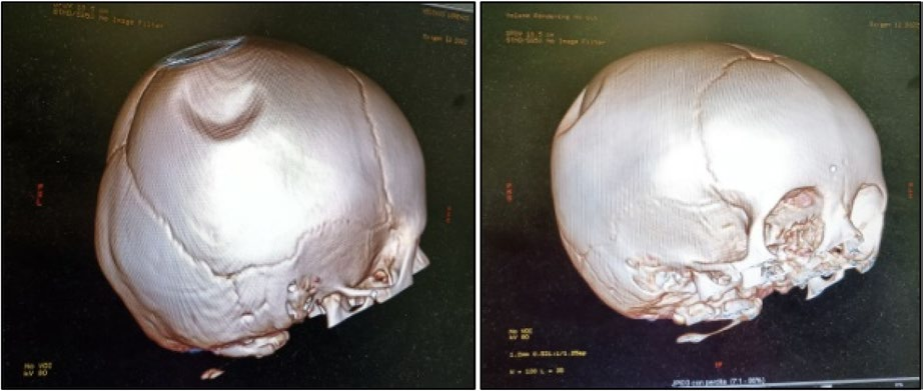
Skull CT images with 3D reconstruction, performed a few hours after the trauma
